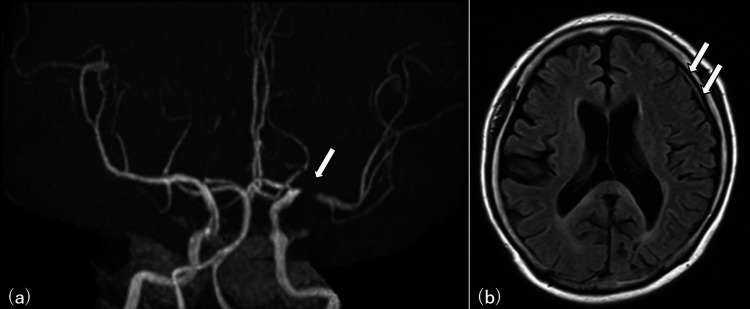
MRI one month prior to admission MRI: magnetic resonance imaging (a) Magnetic resonance angiography (MRA) revealed left middle cerebral artery (MCA) stenosis (arrow). (b) Fluid attenuated inversion recovery (FLAIR) showed dural thickening in the left cerebrum (arrows)
